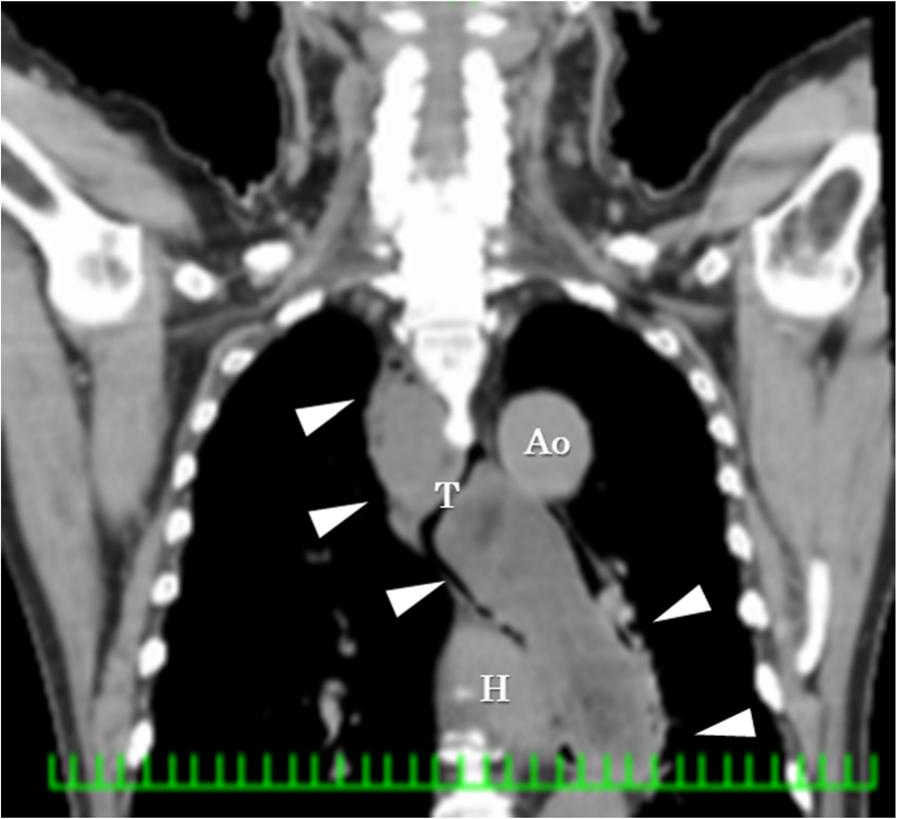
Computed tomography showed giant tumor from the pyriform sinus into the thoracic esophagus intraluminal of the esophagus. The tumor density was mostly low with heterogeneous density in spots (*arrow head*, *T* tumor, *H* heart, *Ao* aorta)
